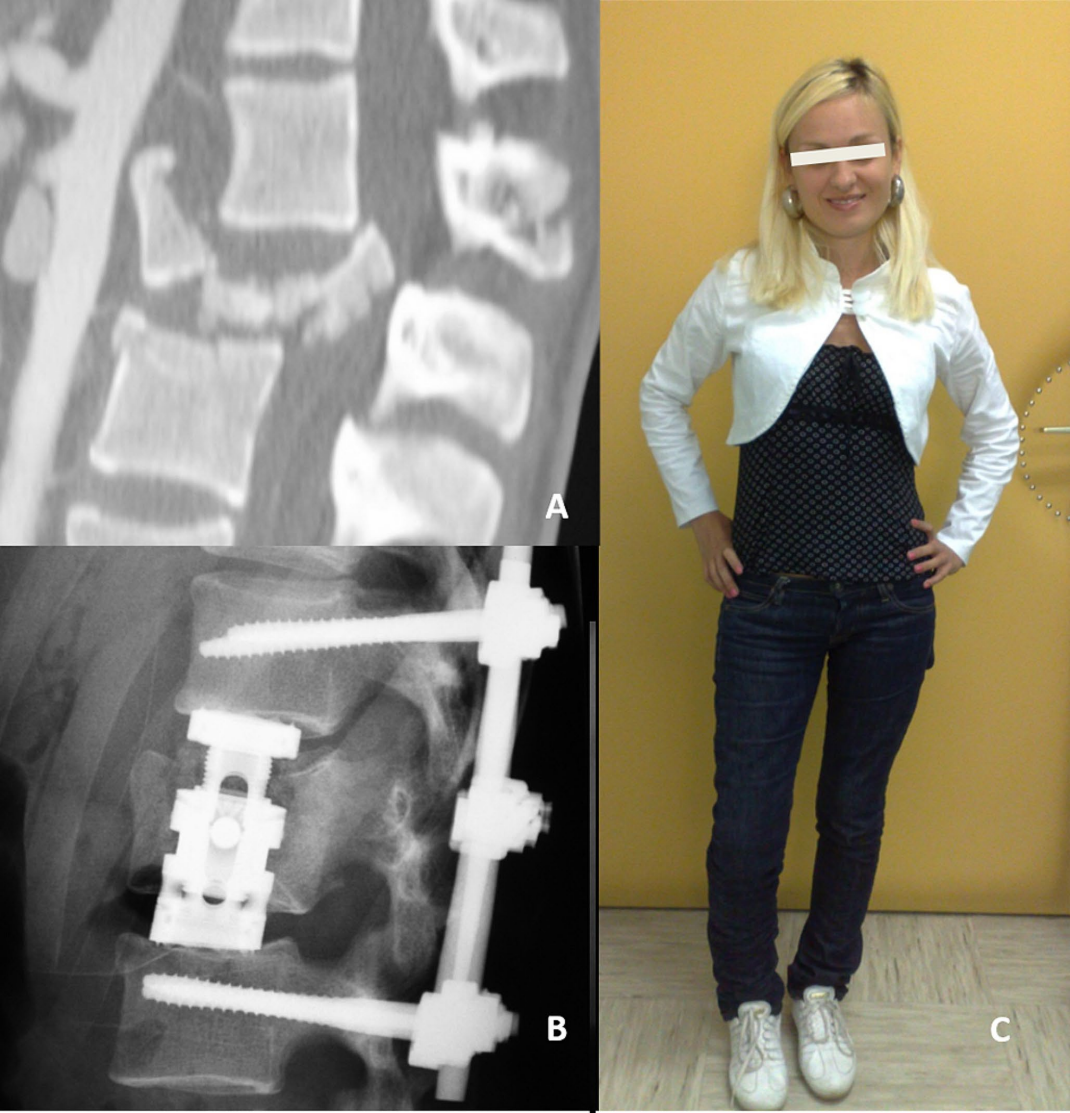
a A case of a polytraumatized patient with complete tSCI and multiple injuries (fracture-dislocation at L1 level, pelvis fracture, both iliac arteries dissection, long bones injuries, etc.) after a suicide jump injury. b The patient first underwent arterial embolisation and vigorous resuscitation in the ICU for 12 h; immediately after circulatory stabilization, a posterior spinal stabilization and realignment was performed with indirect spinal cord decompression (validated by intraoperative CT); two weeks after injury an additional anterior approach was performed for anterior column reconstruction. c One year after injury the patient experienced a complete neurologic recovery
